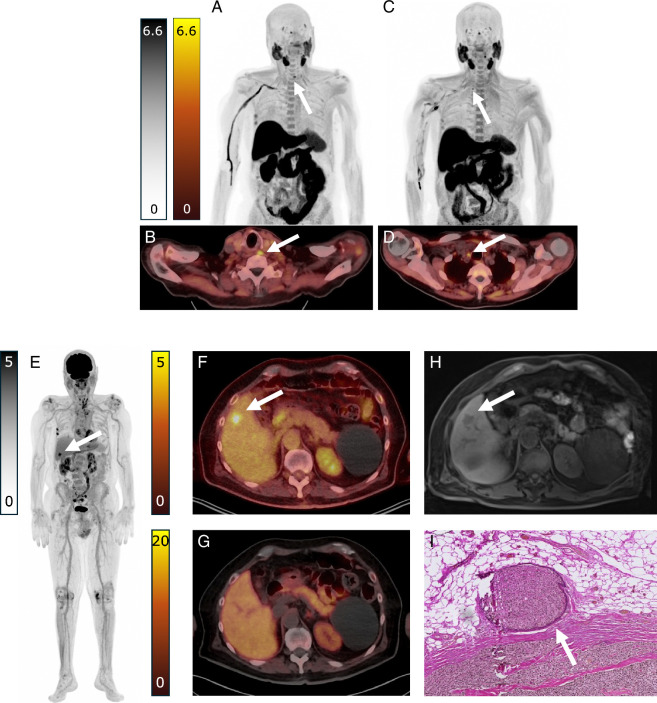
An 80-year-old man with weakness, weight loss of 15 kg in 2 months, hypercalcemia, and hyperparathyroidism underwent [^18^F]F-choline-PET/CT because of suspected parathyroid adenoma. Color bars with standard uptake values (SUV) are presented on the left side of the figures. The maximum intensity projection (MIP) (A) as well as the fused axial slice (B) of the [^18^F]F-choline-PET/CT show a focal uptake dorsal of the left thyroid lobe (marked with arrow) consistent with an imaging-derived diagnosis of a parathyroid adenoma. After surgical removal, pathology assessment confirmed the presumptive diagnosis of parathyroid adenoma. However, the quick recurrence of hypercalcemia, hyperparathyroidism, and severe symptoms prompted another [^18^F]F-choline-PET/CT to rule out missed multiple adenomas. The repeated PET showed a new focal uptake in the right paratracheal area (MIP image C, fused axial slice D, marked with arrow), which was suspected to represent another previously marked parathyroid adenoma or a reactive lymph node. No residual uptake could be seen on the left side. Due to persistently high parathyroid hormone values, an [^18^F]FDG-PET/CT was performed, which showed a focal uptake in the liver (MIP E marked with arrow) in segment V (fused axial slice F , marked with arrow) without [^18^F]F-choline avidity (G). Contrast-enhanced liver MRI confirmed the liver lesion (H, marked with arrow) and detected multiple additional liver metastases, not previously detected on low-dose, nonenhanced CT nor on PET/CT ([^18^F]FDG and [^18^F]F-choline). A concurrent reevaluation of the initial pathology sample revealed a singular vessel invasion (I, marked with arrow), altering the initial diagnosis to parathyroid carcinoma. Postoperative hypercalcemia after resection of a suspected singular parathyroid tumor with elevated parathormone levels can be caused by missed multiglandular disease.^1^ Parathyroid carcinoma is a rare cause for hyperparathyroidism (0.8%–4.6%)^2^ with an incidence per year of around 5.73/10.000.000,^3^ which can result in postoperative persistent hyperparathyroidism due to metastatic disease. Even though parathyroid adenomas can be detected on [^18^F]F-choline-PET/CT with a sensitivity >90%,^4^ imaging of parathyroid carcinoma seems challenging. The usefulness of [^18^F]F-choline-PET/CT for detecting the primary parathyroid carcinoma was reported.^5^ Even metastasized parathyroid carcinomas, with metastases in the skull base,^6^ in the brain,^7^ in the bone, and lungs^8^ could be detected by [^18^F]F-choline-PET/CT. For local recurrence, a case was reported without any differences between [^18^F]FDG-PET/CT and [^18^F]F-choline-PET/CT.^9^ Other authors suggest a dual tracer protocol with [^18^F]FDG and [^18^F]F-choline.^8^ Our case demonstrates 2 relevant findings: first, a solitary lesion on [^18^F]F-choline suspected to represent a parathyroid adenoma can, in rare cases, instead be parathyroid carcinoma. Second, metastatic lesions of parathyroid carcinoma may be missed when relying only on [^18^F]F-choline for imaging. Therefore, dual-tracer protocols should be considered in cases where results are inconclusive.
